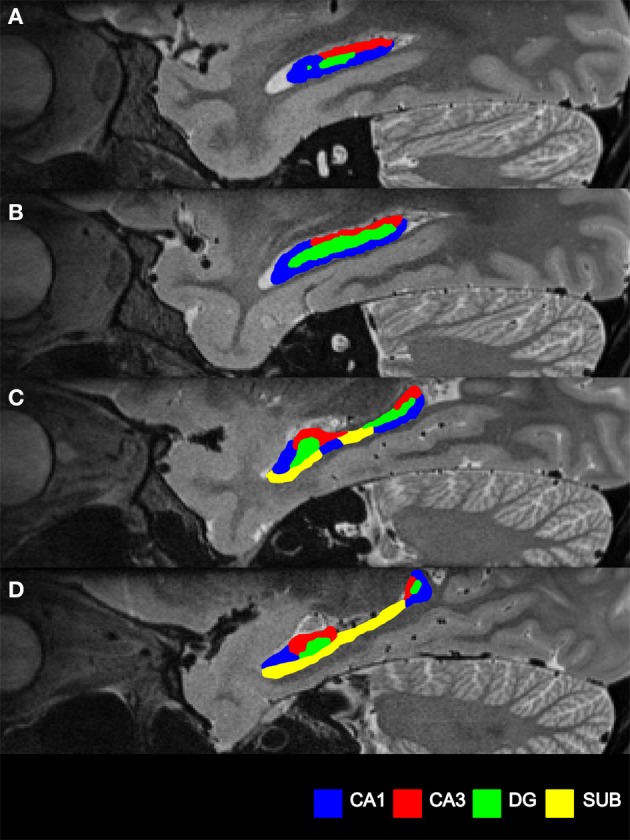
Subfield segmentation in the sagittal plane. (A) Sagittal section through an averaged T2-weighted image of the hippocampus of a participant. Blue indicates CA1, red indicates CA3, the DG in green becoming visible. (B) Proceeding medially through the hippocampus. (C) The body starts to thin and subiculum (yellow) replaces part of CA1. (D) Only head and tail remain at the medial end of the hippocampus, with now most of the subiculum in view.
